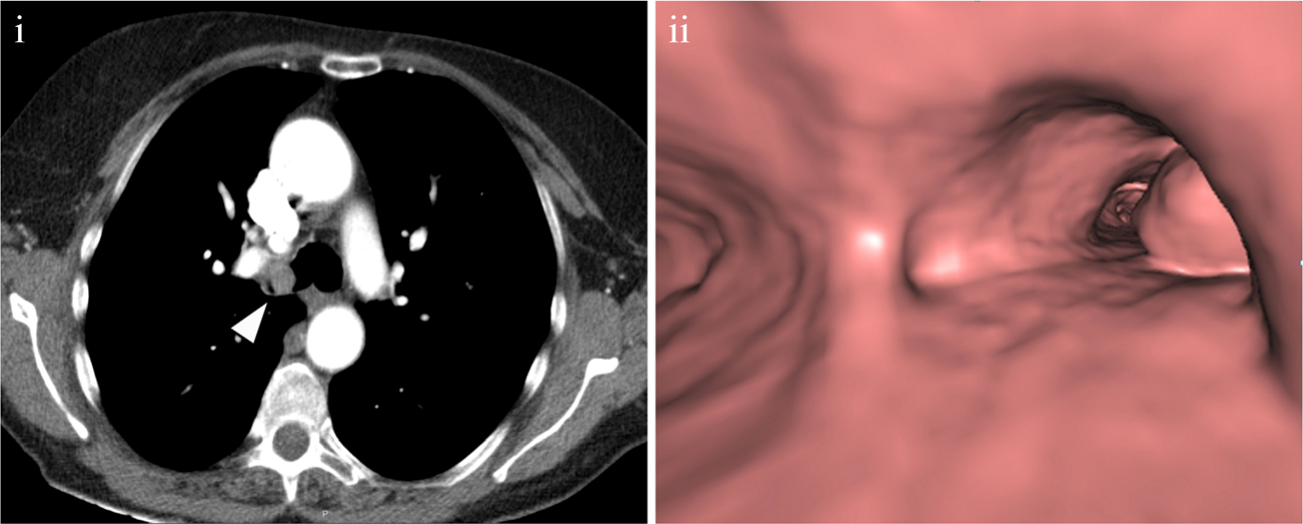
(i) Contrast-enhanced CT scan in a 55-year-old patient showing a nodule within the right main bronchus (*arrowhead*) with avid contrast enhancement, suggestive of a carcinoid tumour. After resection, pathological analysis (not shown) confirmed the diagnosis. (ii) Virtual bronchoscopy depicts the lesion
